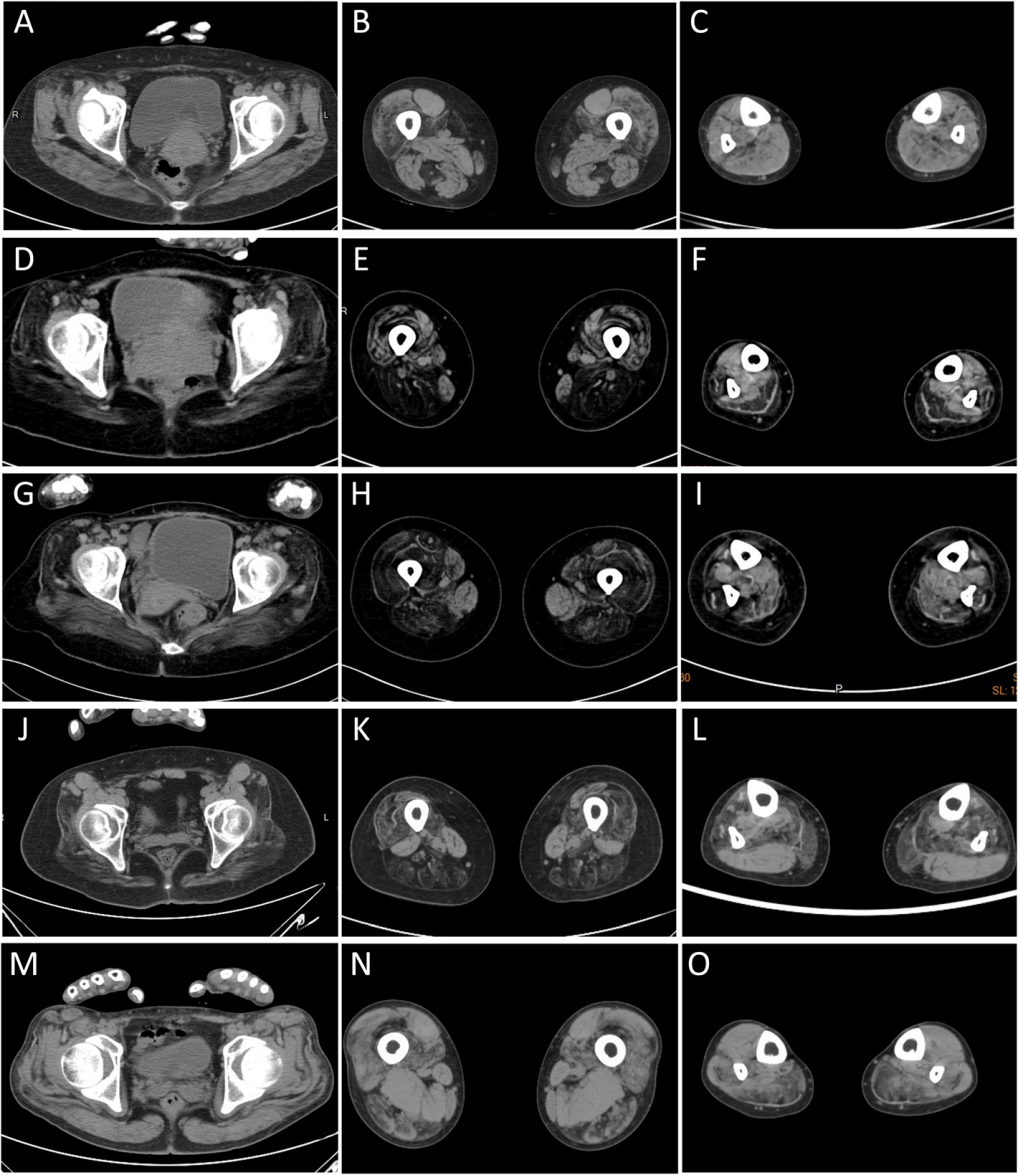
Muscle imaging of LGMD1E, 2A, 2B, 2G, and 2Q. Muscle CT images of 1E revealed most involvement in the S and ST, and less involvement in the VL and VIM at thigh level and the SO, TA, and TP muscles at calf level (a, b, c). Compared with LGMD2A (d, e, f), gluteal muscles were less affected but the TA was more severely involved in LGMD2B (g, h, i). LGMD2G exhibited severe involvement of the gluteus, long head of the BF, AM, ST, SM, SO, medial head of GC, and TA muscles; the short head of BF, AL, S, FD, HL, and lateral head of GC muscles were relatively spared (j, k, l). For LGMD2Q (m, n, o), in the thigh, the AM as well as S and G were relatively hypertrophic and preserved; by contrast, the ST, SM, and QF (except for RF) were markedly affected. At calf level, the GC and P were involved, whereas the TA and EL muscles were preserved and slightly hypertrophic. (AM: adductor magnus; AL: adductor longus; BF: biceps femoris; EL: extensor longus; FD: flexor digitorum; G: gracilis; GC: gastrocnemius; HL: hallucis longus; P: peroneus; QF: quadriceps femoris; RF: rectus femoris; S: sartorius; SO: soleus; ST: semitendinosus; SM: semimembranosus; TA: tibialis anterior; TP: tibialis posterior; VL: vastus lateralis; VIM: vastus intermedius)
